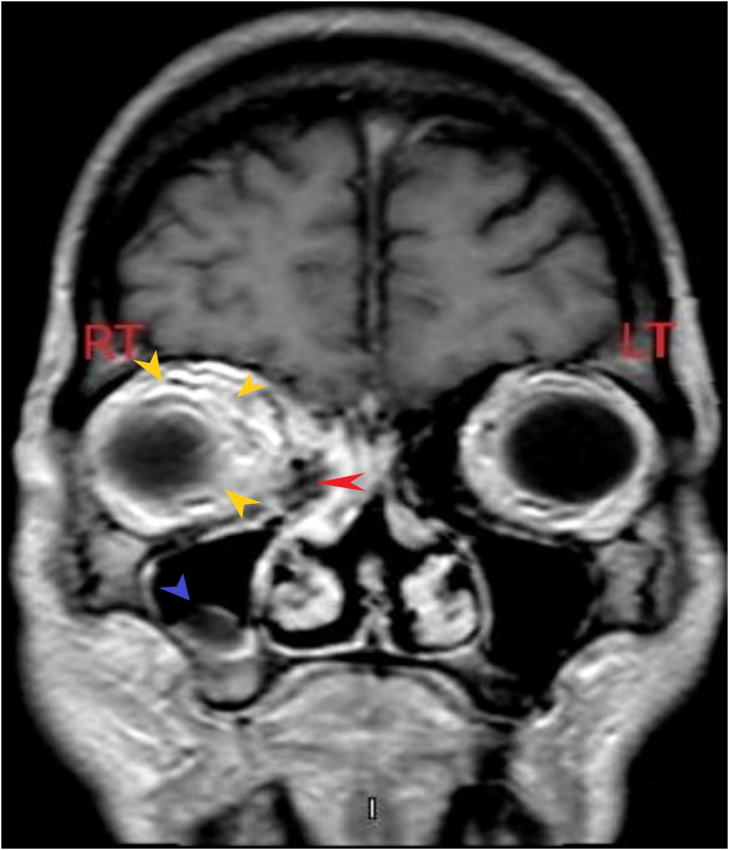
MRI Head with contrast (T1 weighted coronal view) scan showing enhancement at the right periorbital area (yellow arrow-heads) suggestive of periorbital cellulitis, along with opacification at right ethmoidal (red arrow-head) and right maxillary (blue arrow-head) sinuses. MRI: Magnetic Resonance Imaging. R.T.: Right, L.T.: Left.
